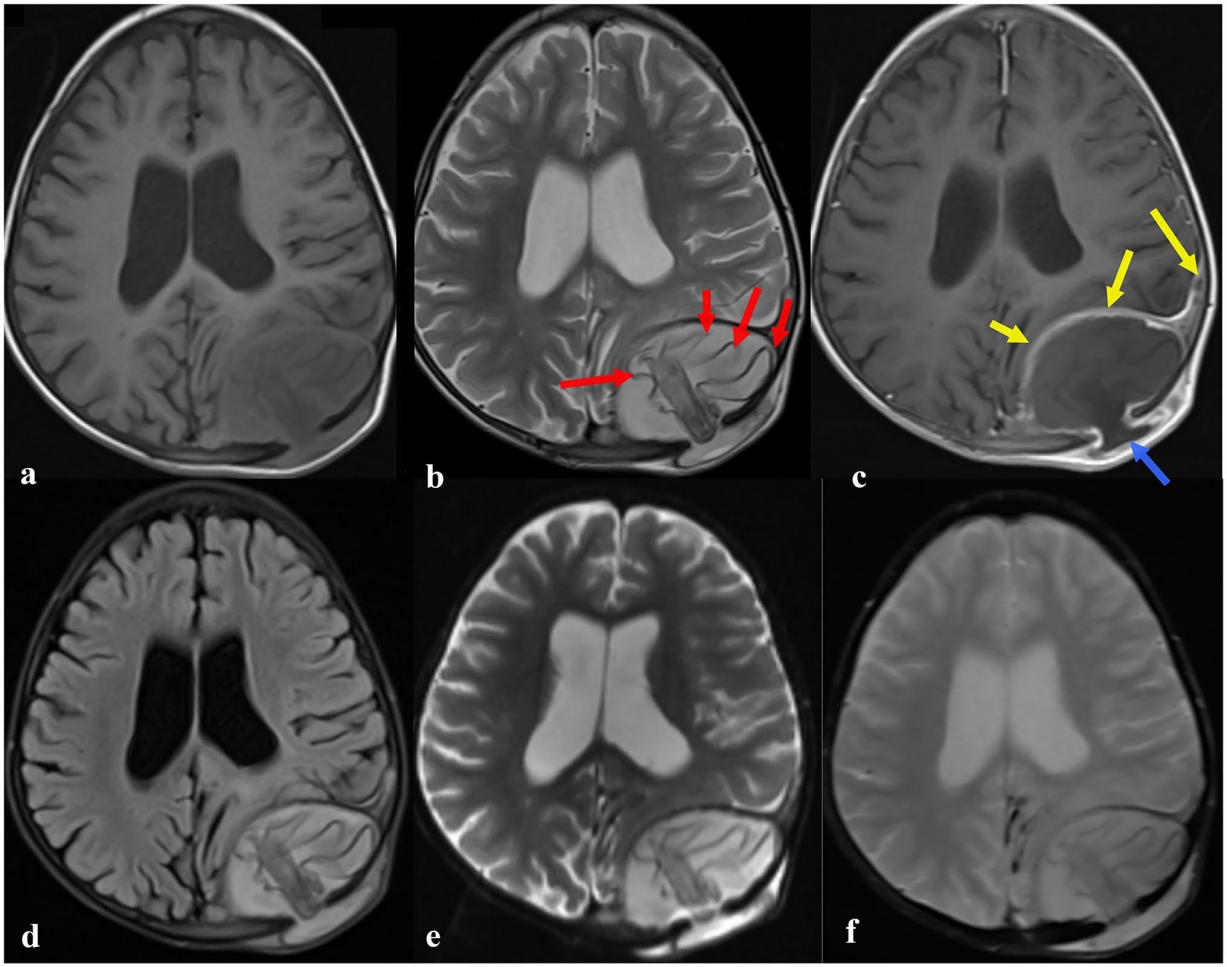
Axial brain MRI showing a well-limited extra-axial lesion of heterogeneous liquid signal, in hyposignal on T1-weighted sequences (a) and hypersignal on T2-weighted sequences (b) with floating membranes (b, red arrows) and a wall in hyposignal on T2-weighted sequence. Note the peripheral rim enhancement as well as of the adjacent dura mater after gadolinium chelates injection (c, yellow arrows). Note the absence of edema surrounding the cyst on the T2-fluid attenuated inversion recovery sequences (FLAIR) (d) as well as the absence of any abnormal signal on diffusion weighted sequences (e) and gradient echo sequences (f)
